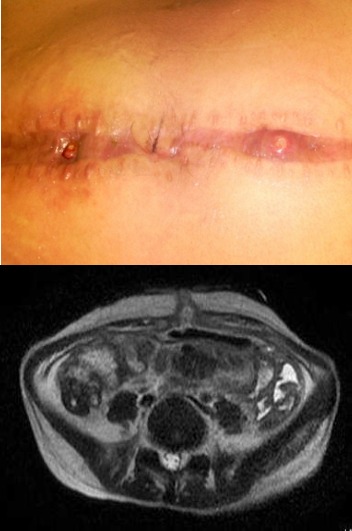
Two sinus tracts in the midline of abdominal wall and abdominal computed tomography showing the sinus tract
